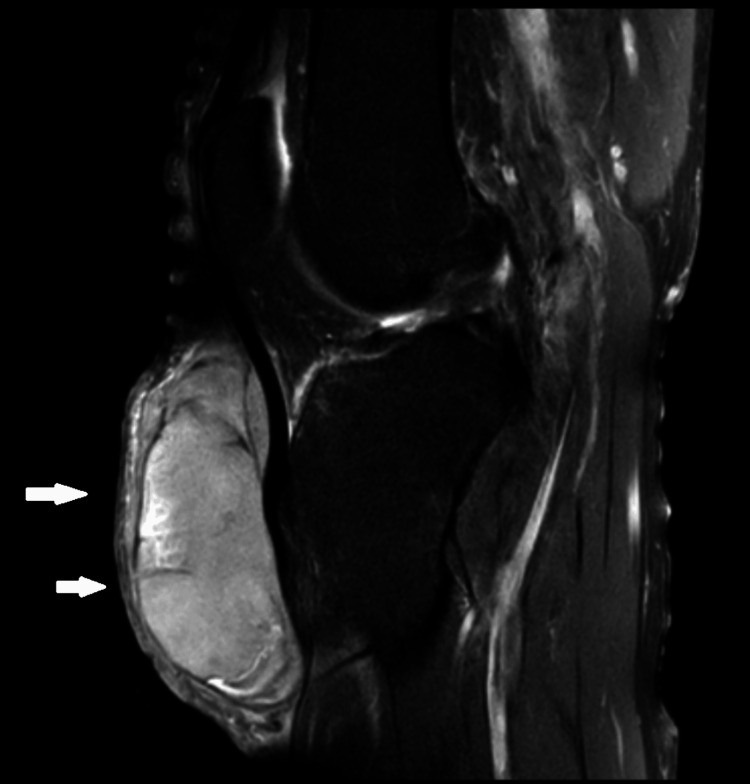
Sagittal MRI section showing the tumor mass This sagittal section shows a well-defined mass, with regular contours, in intermediate T2 hypersignal, in T1 hyposignal, and in diffusion hypersignal, which enhances heterogeneously after a gadolinium injection. The arrows show the location of the mass in the subcutaneous soft tissue of the right knee.
